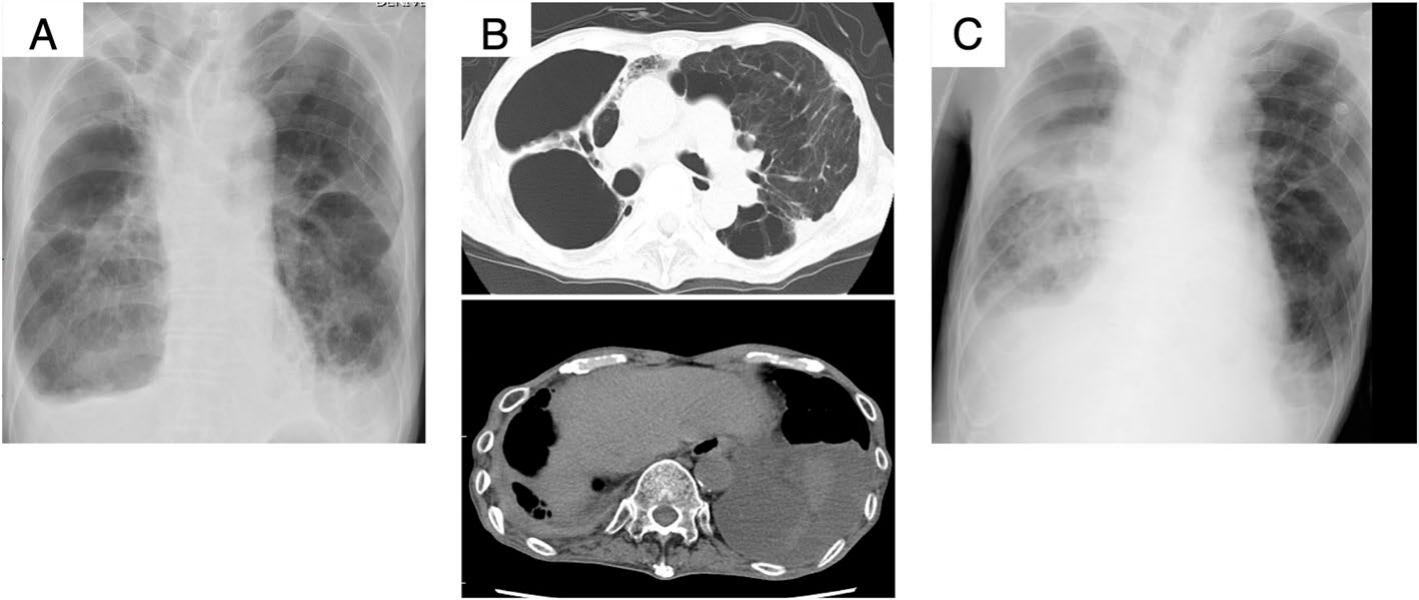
Chest radiography and computed tomography findings. Chest radiography (a) and chest computed tomography (b) findings obtained at hospitalization. Chest radiography findings obtained on the 37 ^th^ disease day (c)
